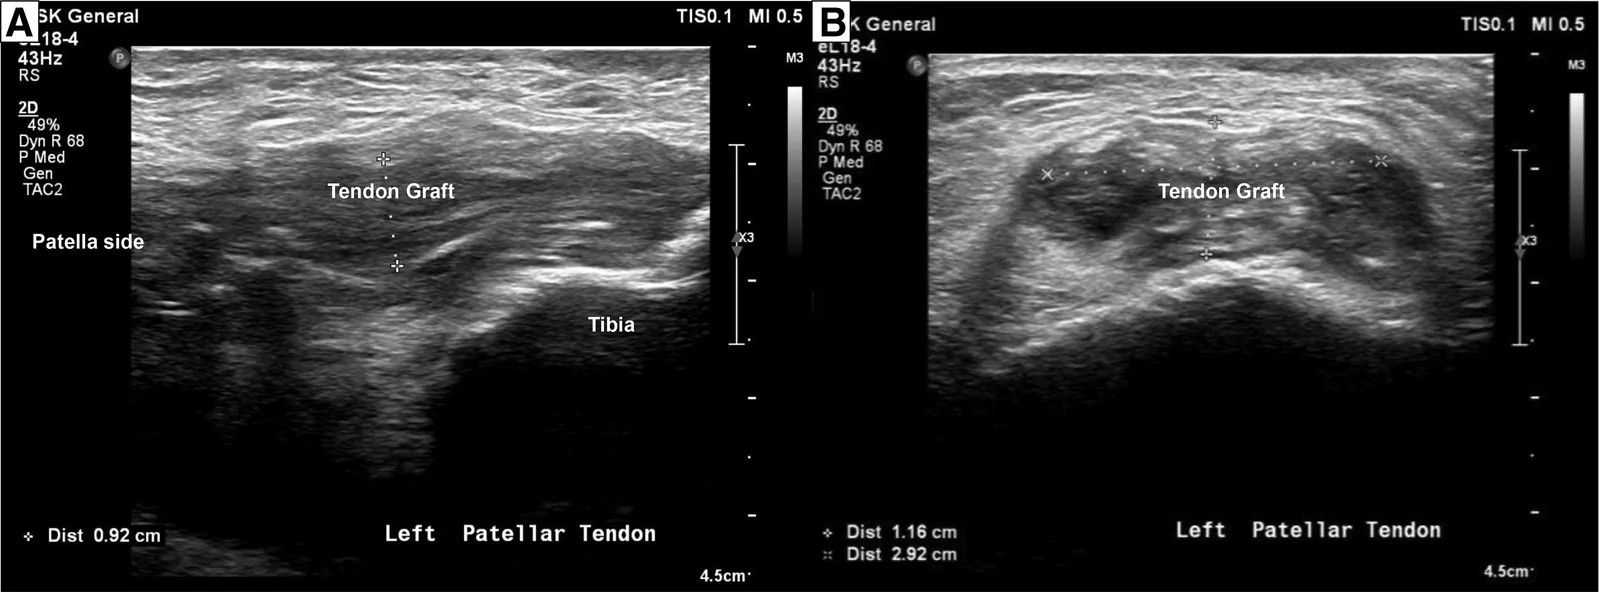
One-year postoperative ultrasonography of the left knee. (A) Longitudinal view along the long axis of the extensor mechanism demonstrating structural continuity of the reconstructed peroneus longus autograft from the patellar insertion to the tibial attachment. (B) Transverse view at the mid-substance level showing a well-organized, thick graft construct with preserved structural integrity and no evidence of intratendinous disruption.
